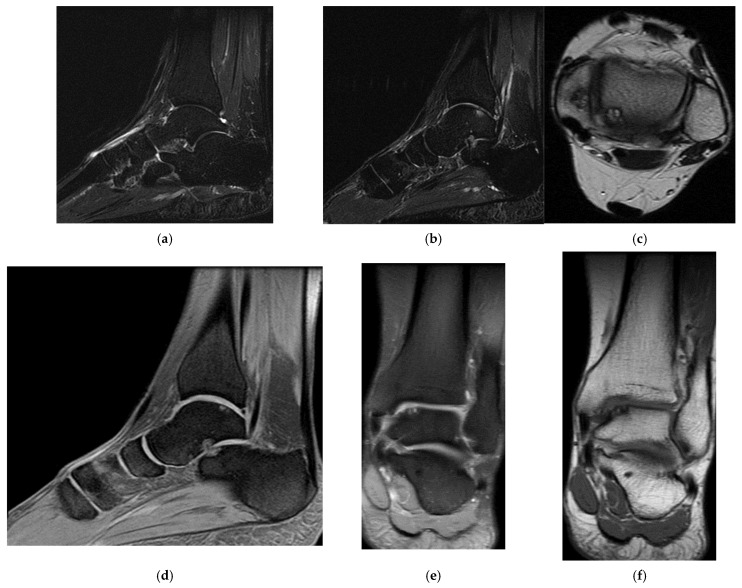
(a) Ankle MRI: sagittal stir acquisition, (b) ankle MRI: sagittal stir acquisition, (c) ankle MRI: axial T2 acquisition, (d) ankle MRI: sagittal T2 fat sat acquisition, (e) ankle MRI: coronal PD fat sat acquisition, (f) ankle MRI: coronal T1.
